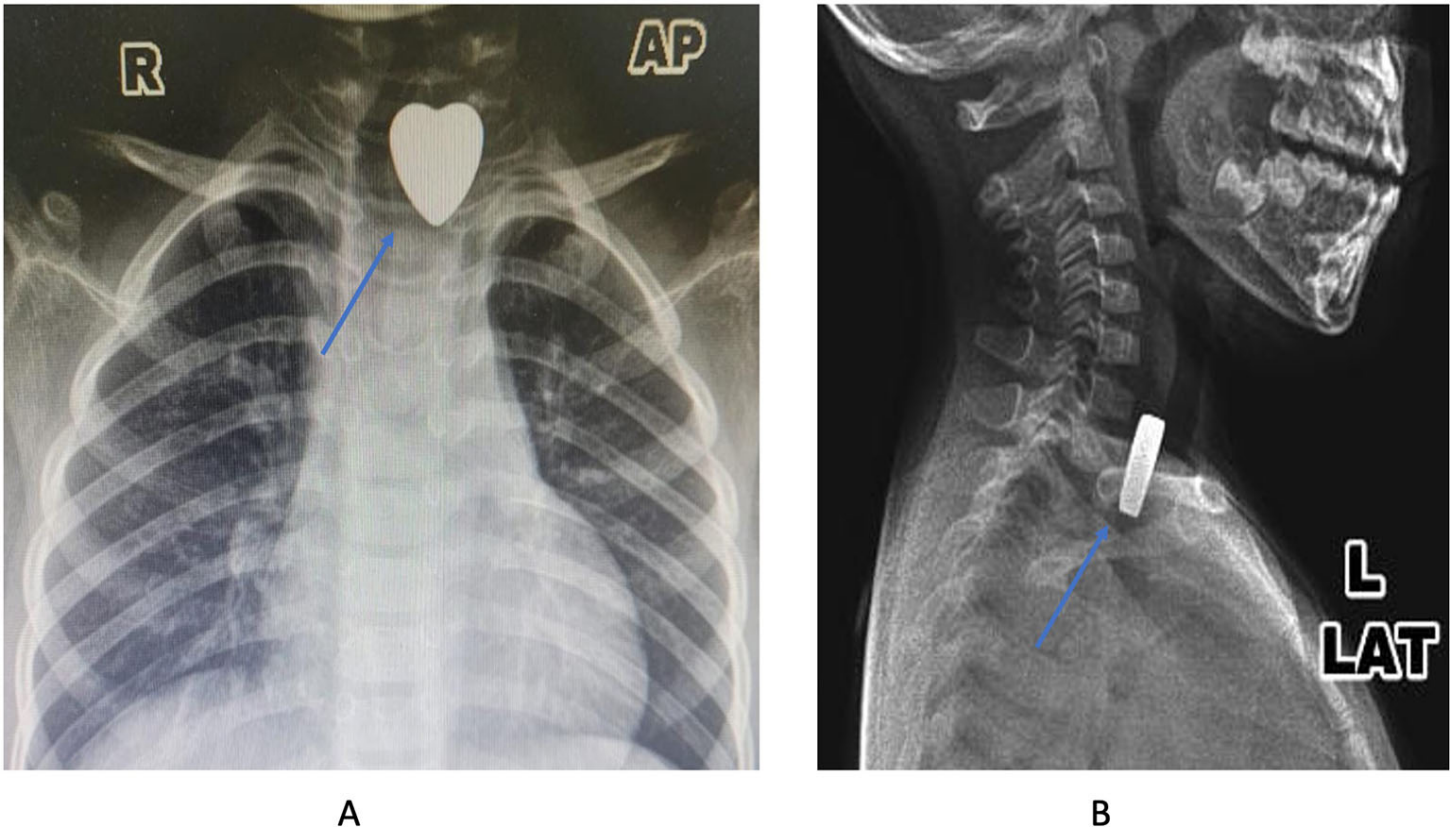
The X-ray images showed the metallic density with the heart shape (blue arrow) (A: anterior-posterior projection; B: lateral projection).
